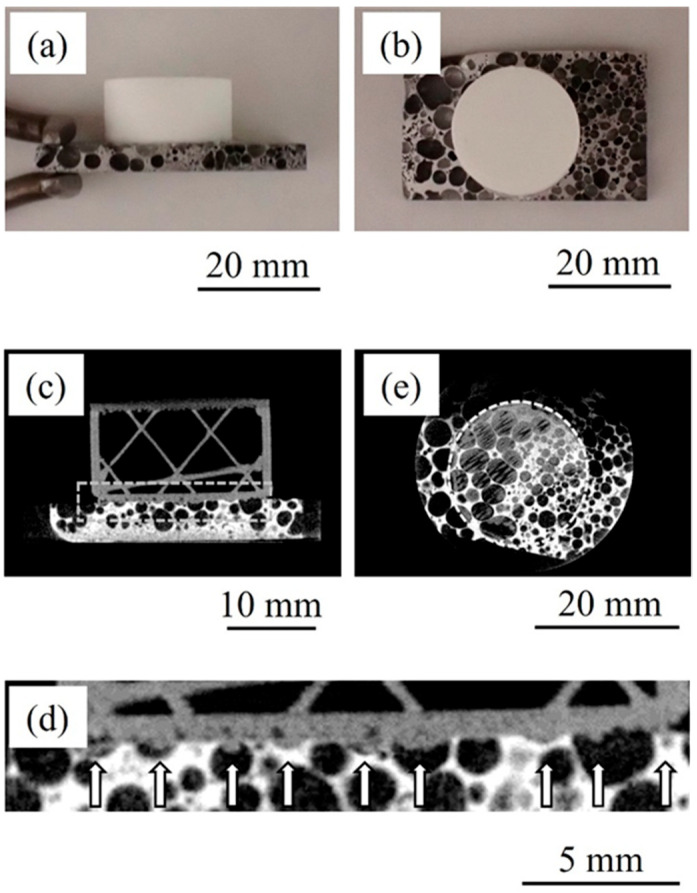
Bonded sample in which resin was directly printed on heated aluminum foam. (a) Side view. (b) Top view. (c) X-ray CT image viewed from side. (d) Enlarged image of dotted box in (c). White arrows show where the resin penetrated into pores. (e) X-ray CT image viewed from above.
